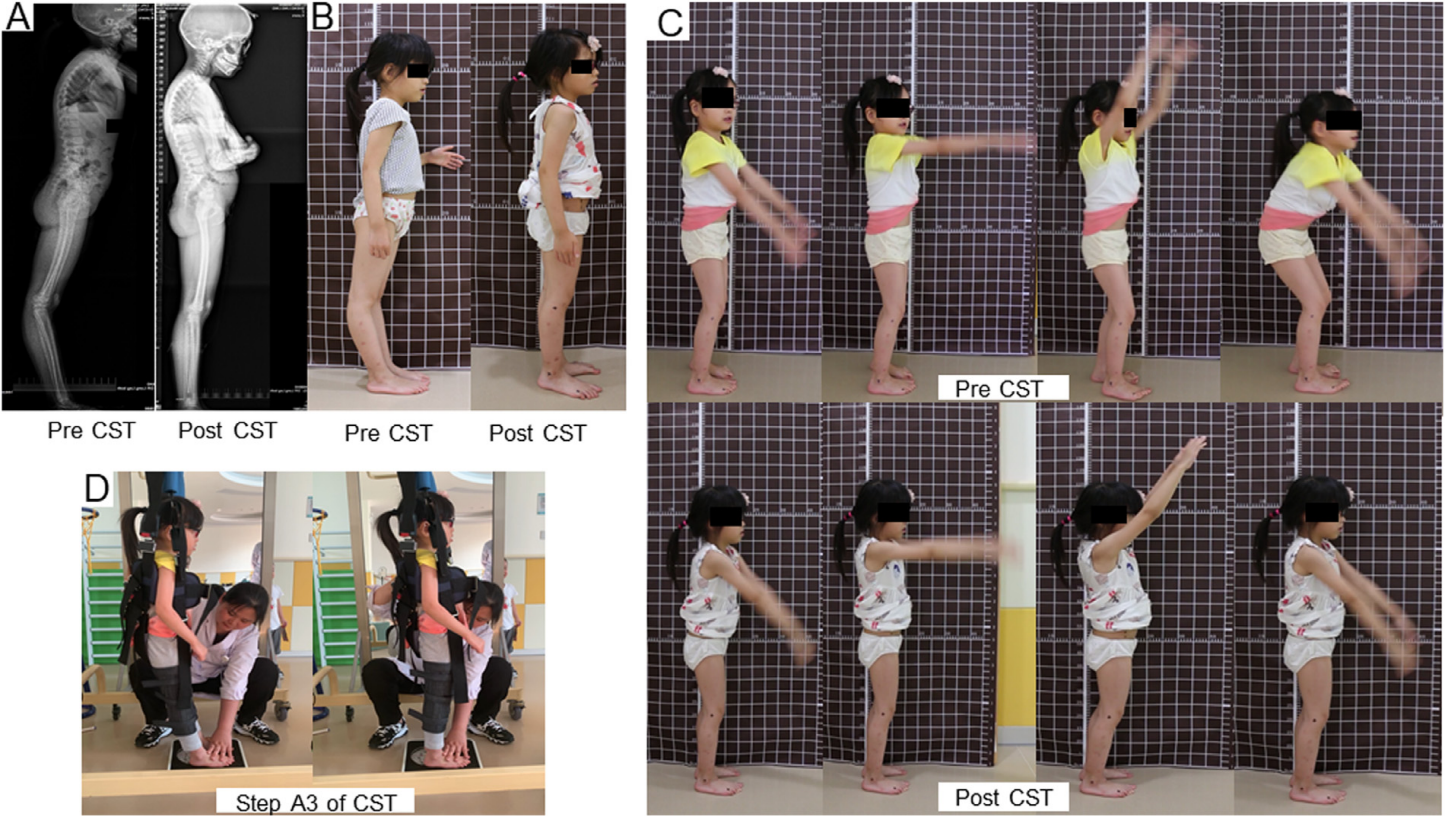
Static and dynamic postural alignment before and after CST. (A) Lateral plain radiographs of the whole body showed that the patient had hyperkyphosis, lumbar hypolordosis, posterior pelvic tilt, and knee hyperextension. After the treatment, knee hyperextension recovered. (B) The static standing posturographies from the right-side view. (C) Dynamic stability alignment of lower limbs with arms waving overhead before and after CST showing that the patient could keep knee straight unconsciously after CST. (D) Step A3 of CST. The physical therapist fixes the patient's feet and helps the patient swing her pelvis in sagittal plane and then induces her to maintain the position.
